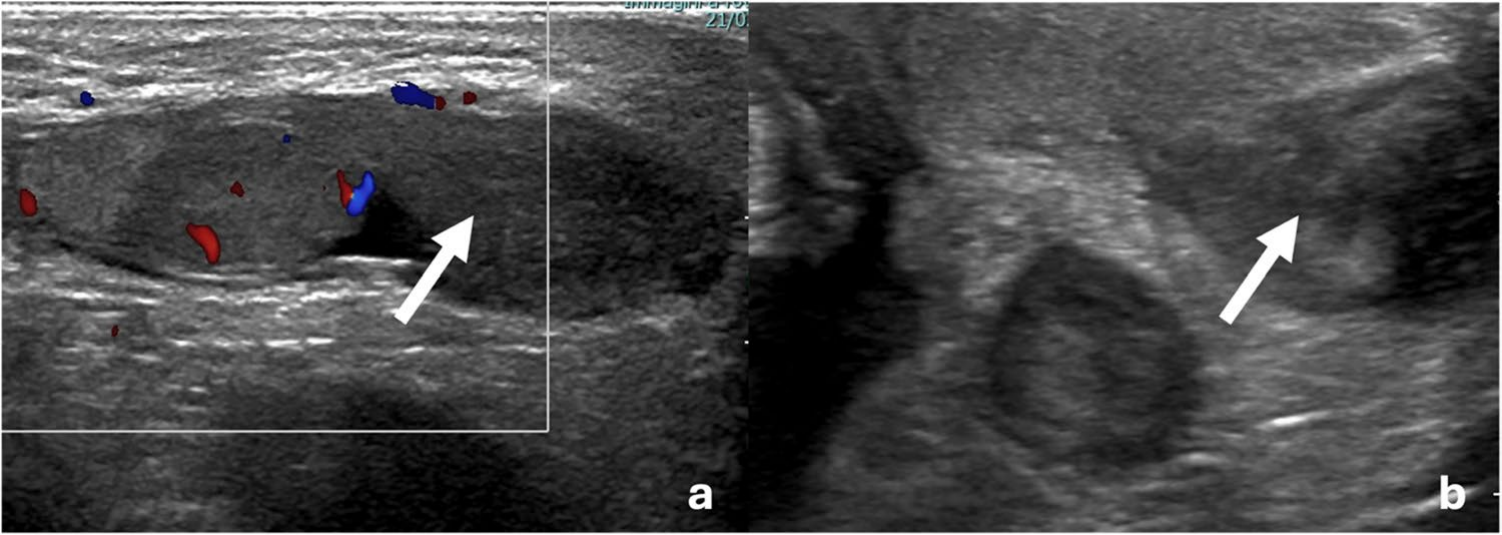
Longitudinal (a) and transversal (b) view of the testicular ultrasound performed at day 4 of life, showing the scrotal hematoma (*white arrow*)
